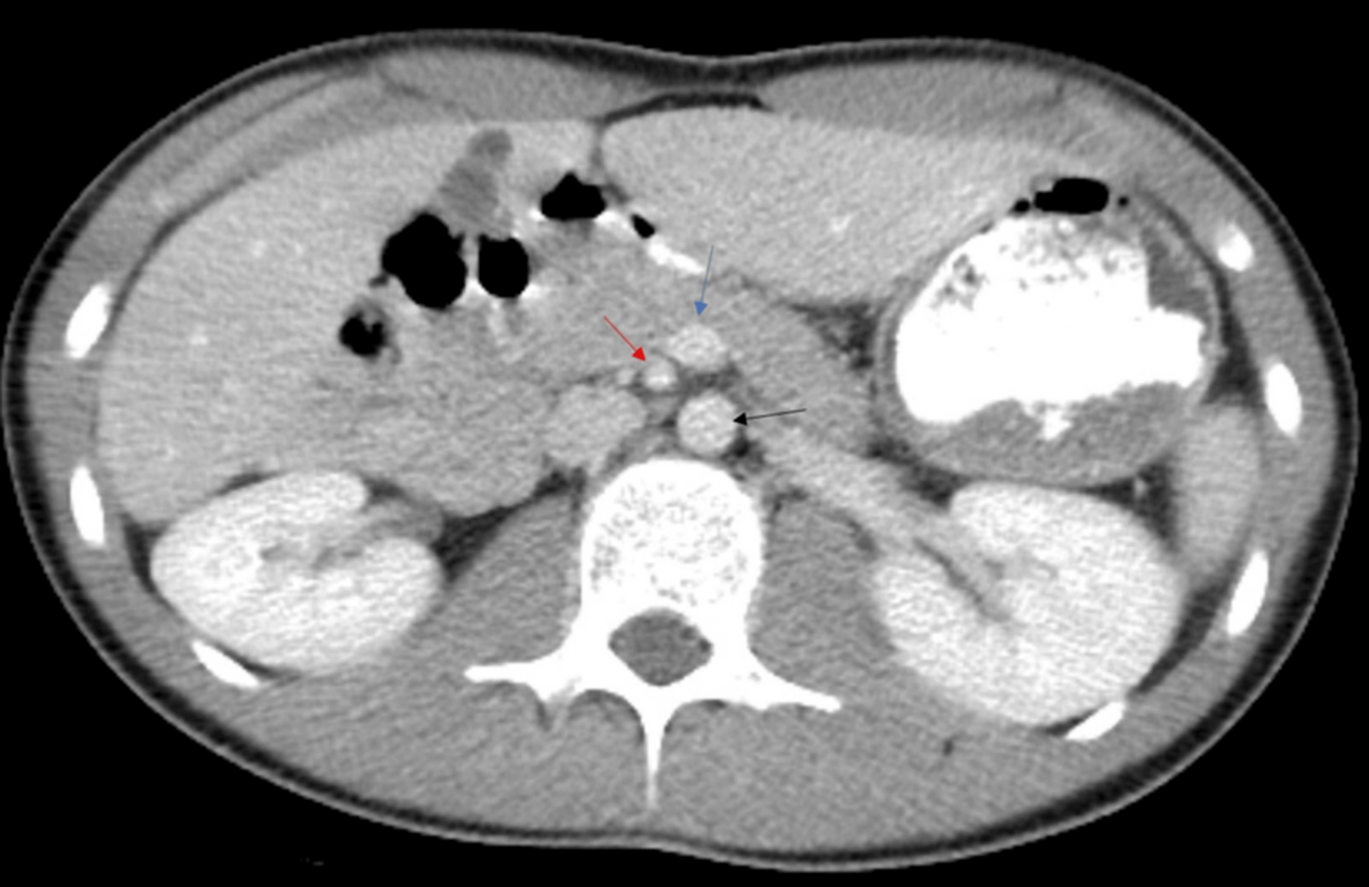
Axial contrast-enhanced CT image of the upper abdomen This image reveals a reversal of the normal relationship of the superior mesenteric artery (SMA, red arrow) and superior mesenteric vein (SMV, blue arrow), with SMV lying on the left side of the SMA. Note that the duodenum is not seen crossing between the aorta (black arrow) and the SMA (red arrow).
